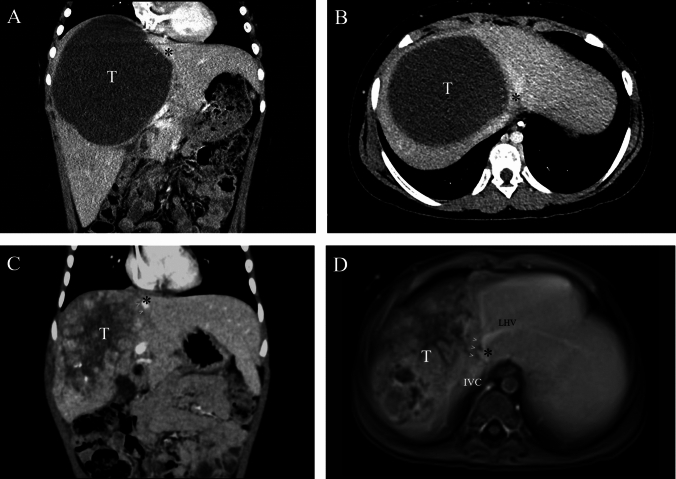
a, b CT scan showing the coronal and axial view of patient 3; > arrows showing tumor contact. c, d CT scan (coronal) and MRI (axial) of patient 14; > arrows showing tumor contact. *CT* computed tomography, *MRI* magnetic resonance imaging, *T* tumor, *IVC* inferior vena cava, *LHV* left hepatic vein, * common trunk of the middle and left hepatic veins
